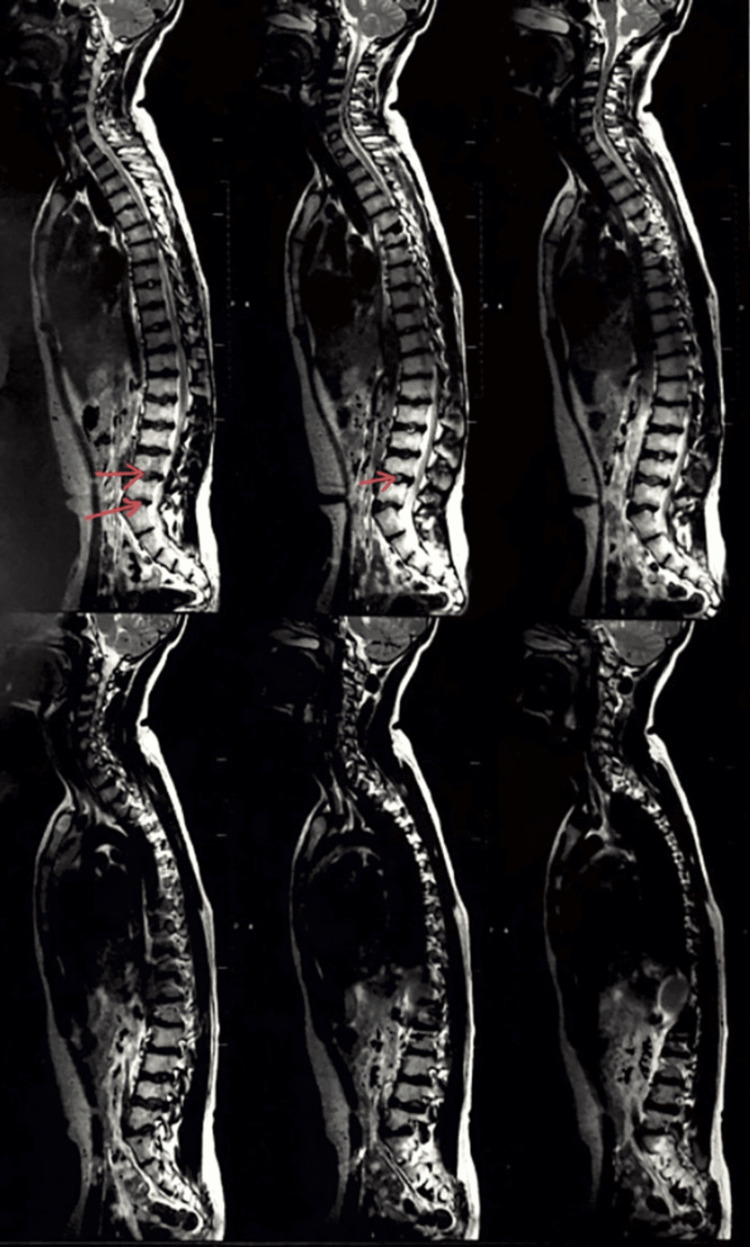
MRI showing the shmorl's nodules between the vertebral discs These red arrows show the schmorl''s nodules between the vertebral discs Image Credit: Author: Arpit Jain
